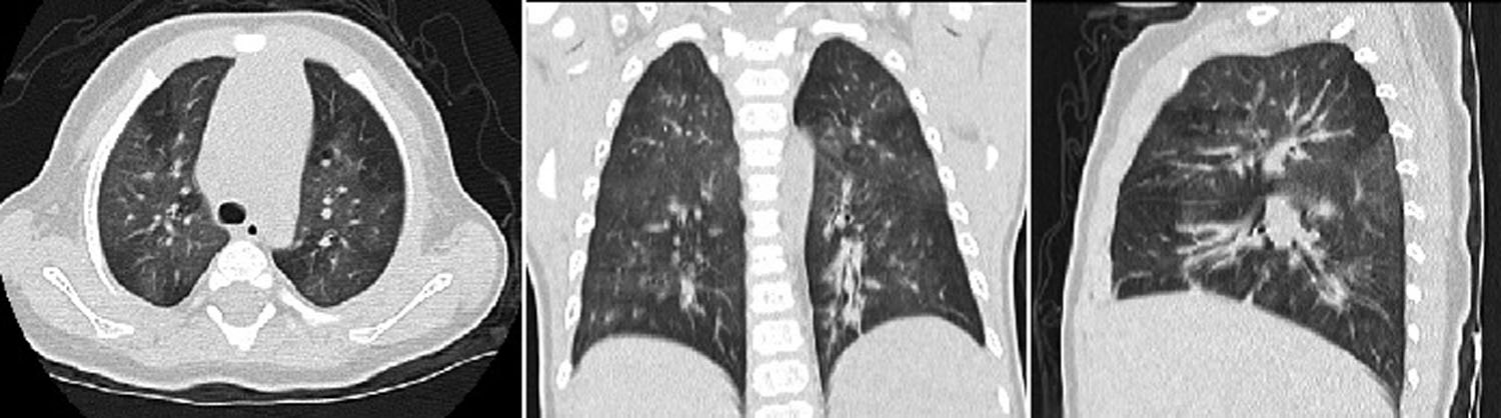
The chest computed tomography of the patient 7 showed mosaic perfusion and air trapping for bronchiolitis obliterans at the follow-up.
